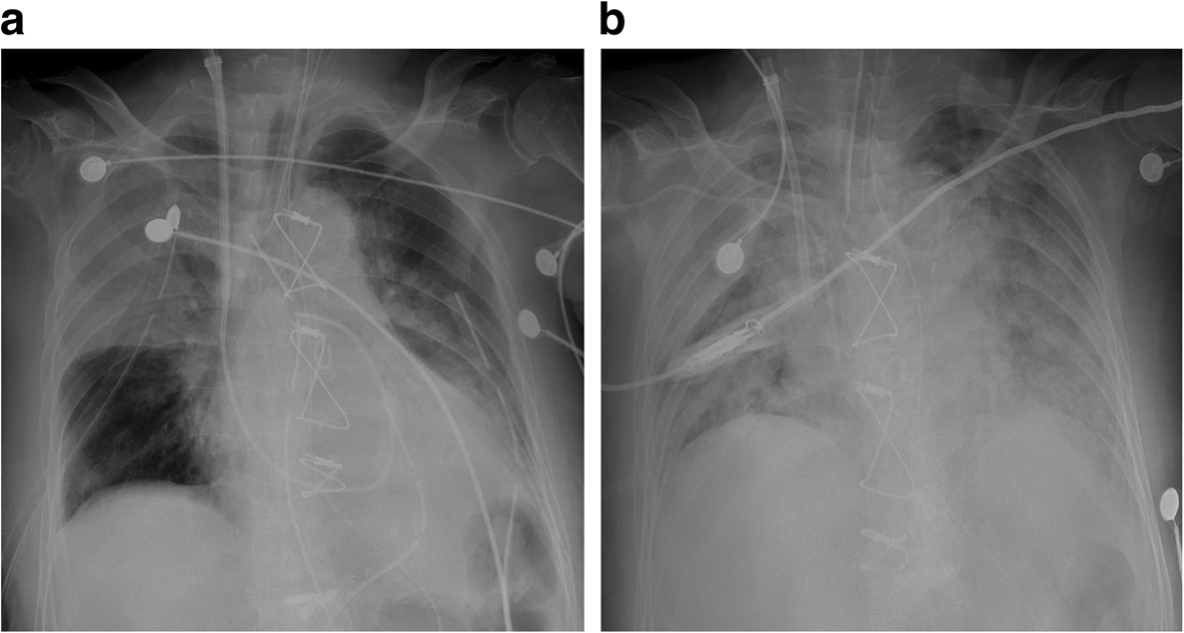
a Postoperative chest roentgenogram showing abrupt haziness of the right upper lung field on day 4 after surgery and (b) rapid deterioration of adult respiratory distress syndrome (ARDS) on day 8 after surgery
